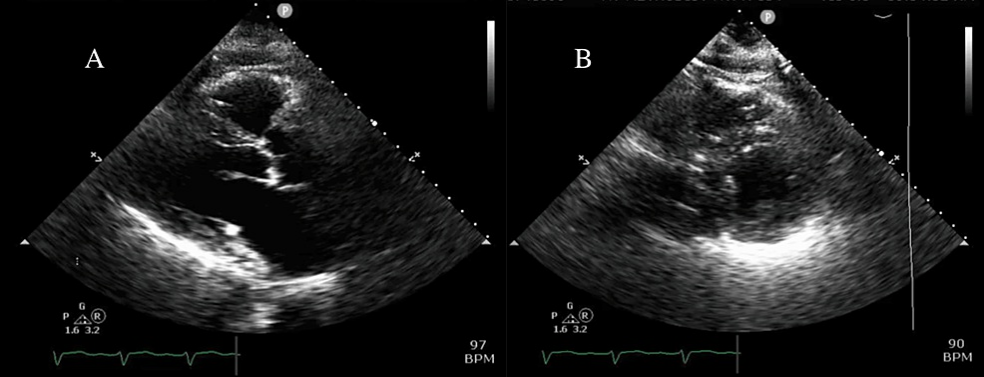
Transthoracic echocardiogram Parasternal long axis view (A) and parasternal short axis view (B) demonstrating moderate concentric left ventricular hypertrophy, a moderately dilated left ventricle, and an interventricular septal diameter of 1.2 cm.
